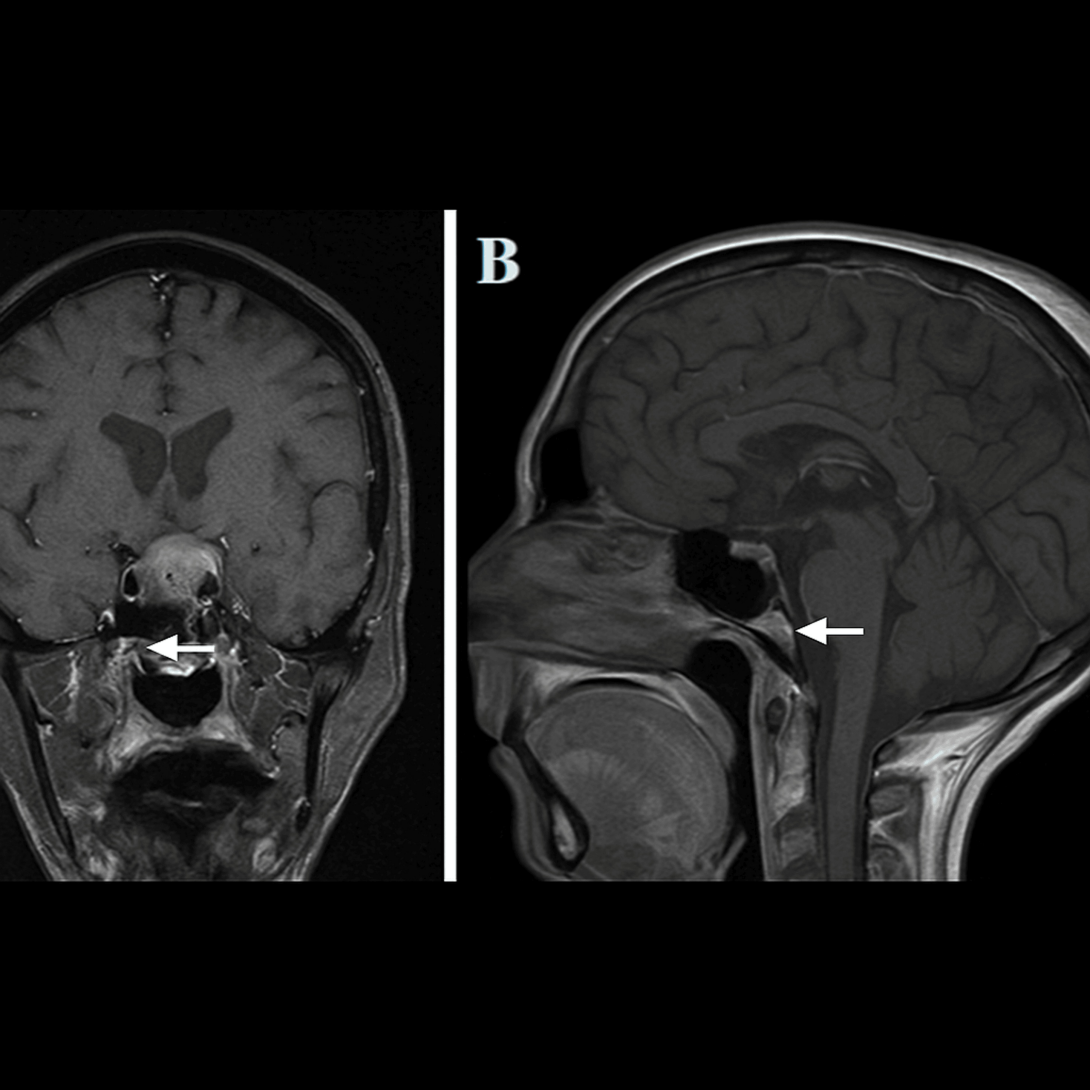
MRI pituitary post-contrast revealed a heterogeneously enhancing pituitary lesion extending into the suprasellar compartment (A) Preoperative T1 MRI with contrast – coronal view. (B) Preoperative T1 MRI with contrast – sagittal view MRI: magnetic resonance imaging
